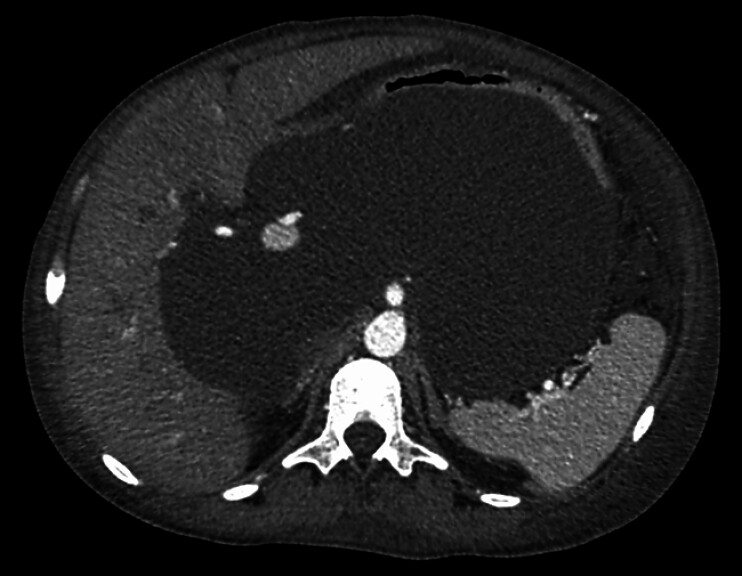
Initial abdominal CT scan shows a well-defined, encapsulated peritoneal pseudocyst in the lesser sac, causing compression of the posterior gastric wall.
